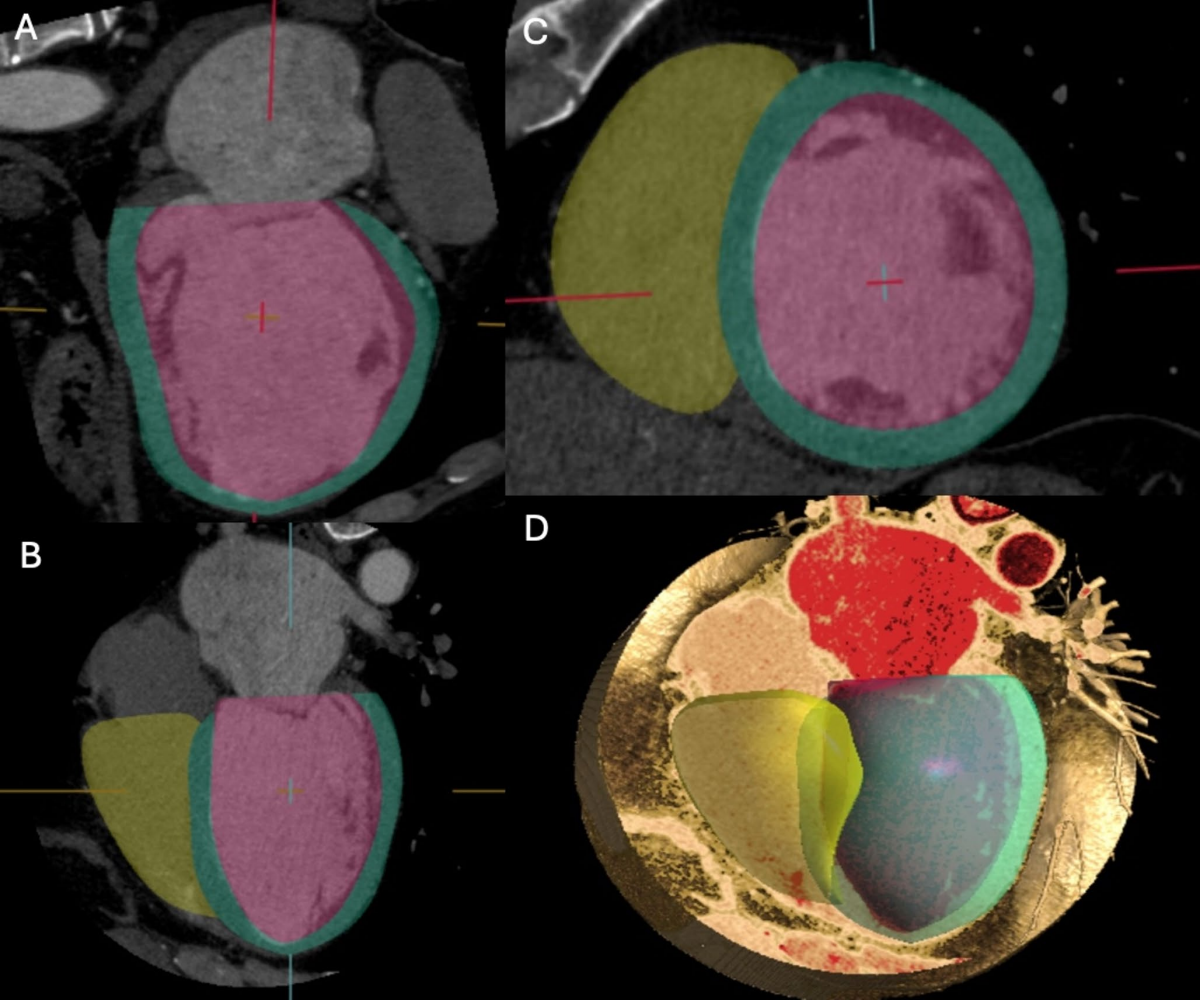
Practical example of left and right ventricular volume and function assessment using computed tomography. Endocardial and epicardial borders of the left ventricle are delineated with red and green contours, respectively, in both long-axis (Panels A and B) and short-axis views (Panel C). Yellow lines in Panel C indicate the endocardial borders of the right ventricle. Panel D shows a volumetric rendering of a four-chamber view with an endoluminal perspective. Images were post-processed using Circle CVI software
